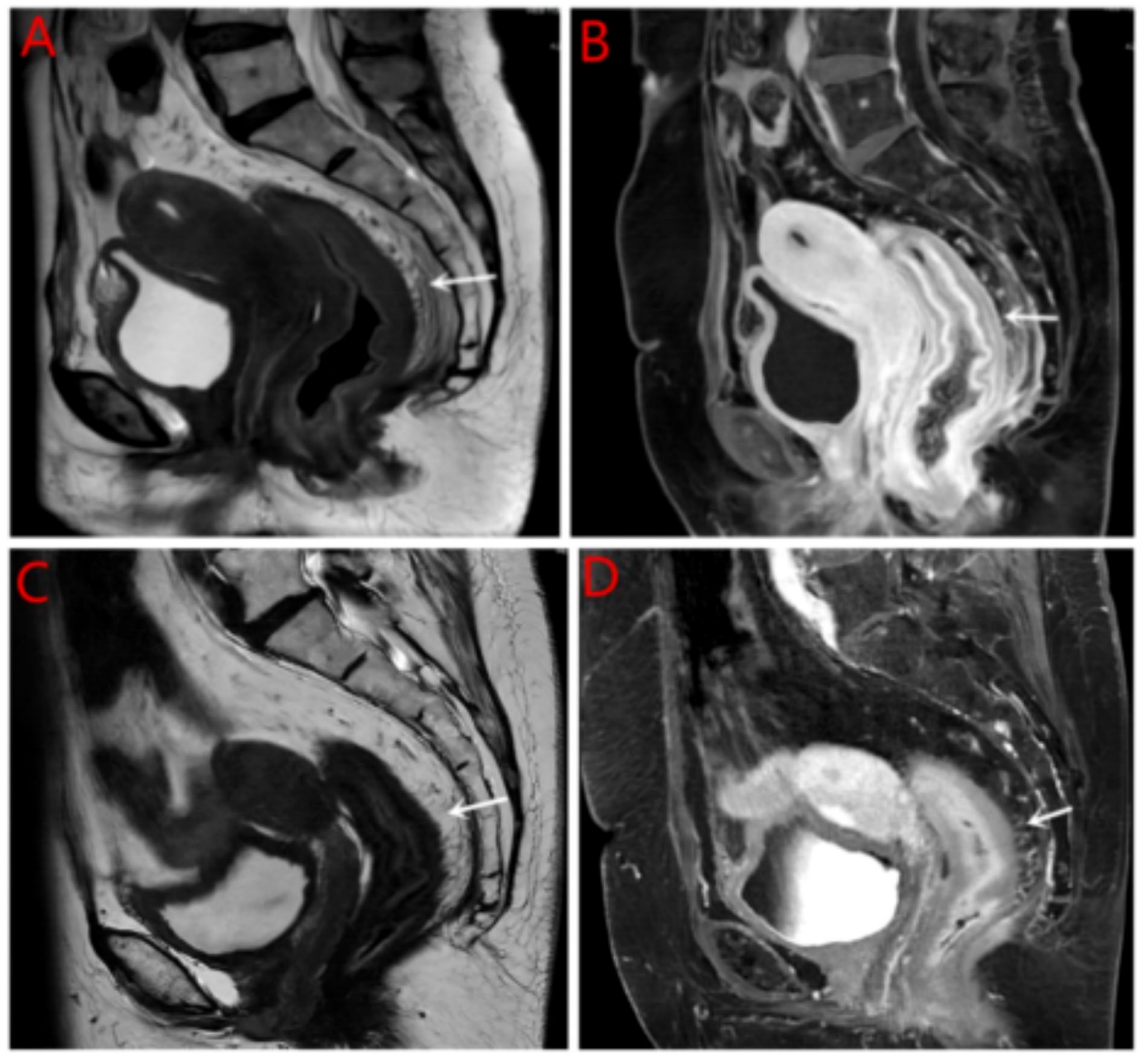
MRI rectal wall. (A) Pretreatment with MRI T2W1 imaging of the rectal wall; (B) Pretreatment with enhanced MRI imaging of the rectal wall; (C) Post treatment with MRI imaging of the rectal wall; (D) Pretreatment with enhanced MRI imaging of the rectal wall.
